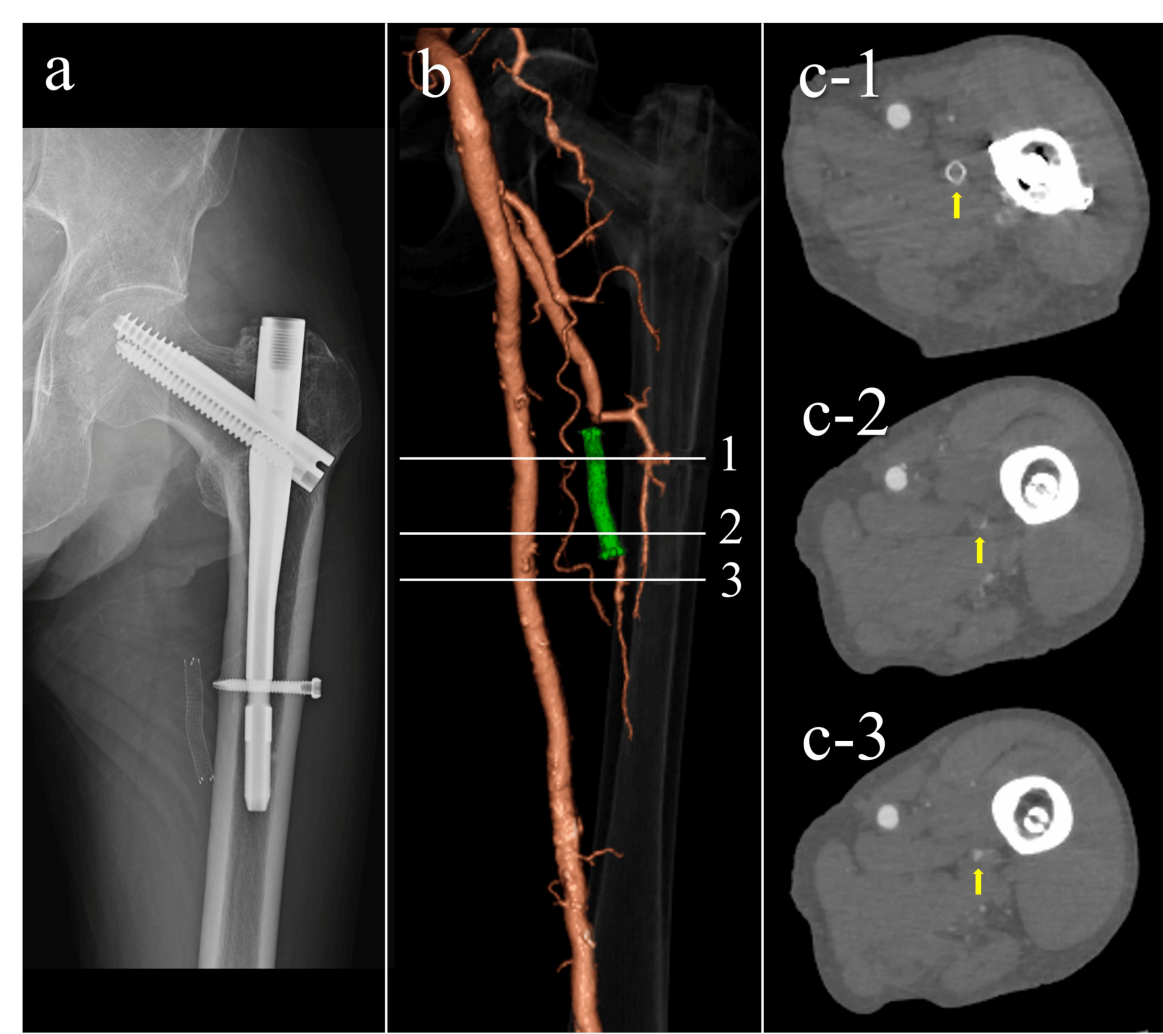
Follow-up imaging at three years postoperatively (a) Plain radiograph showing no fracture or migration of the stent. (b) Three-dimensional computed tomography angiography showing the deployed stent graft (green) in the deep femoral artery. A thin peripheral vessel is visible distal to the stent, indicating minimal preserved flow. Regions 1 to 3 correspond to the cross-sectional slices shown in c-1 to c-3. (c) Axial contrast-enhanced CT images at three levels (1 to 3 in b), demonstrating near-complete occlusion of the stent lumen (yellow arrows) without evidence of lower limb ischemia
